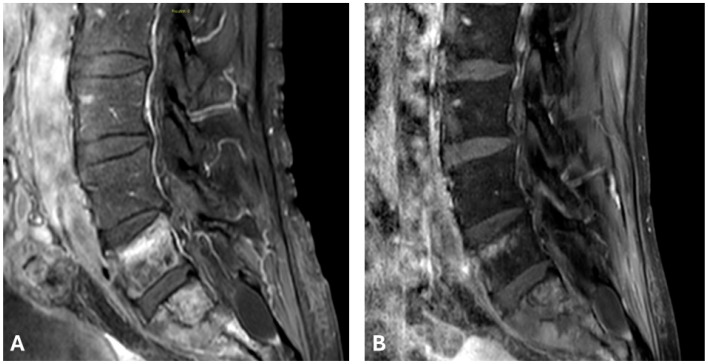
Contrast-enhanced T1-weighted fat-saturated MRI sequences at baseline show contrast uptake in lesions at the levels of L5, S1 and S2, as well as in the extracompartmental component of S1 (A). The same sequences, at the 3-month follow-up, display a reduction in vertebral and extracompartmental contrast uptake (B).
